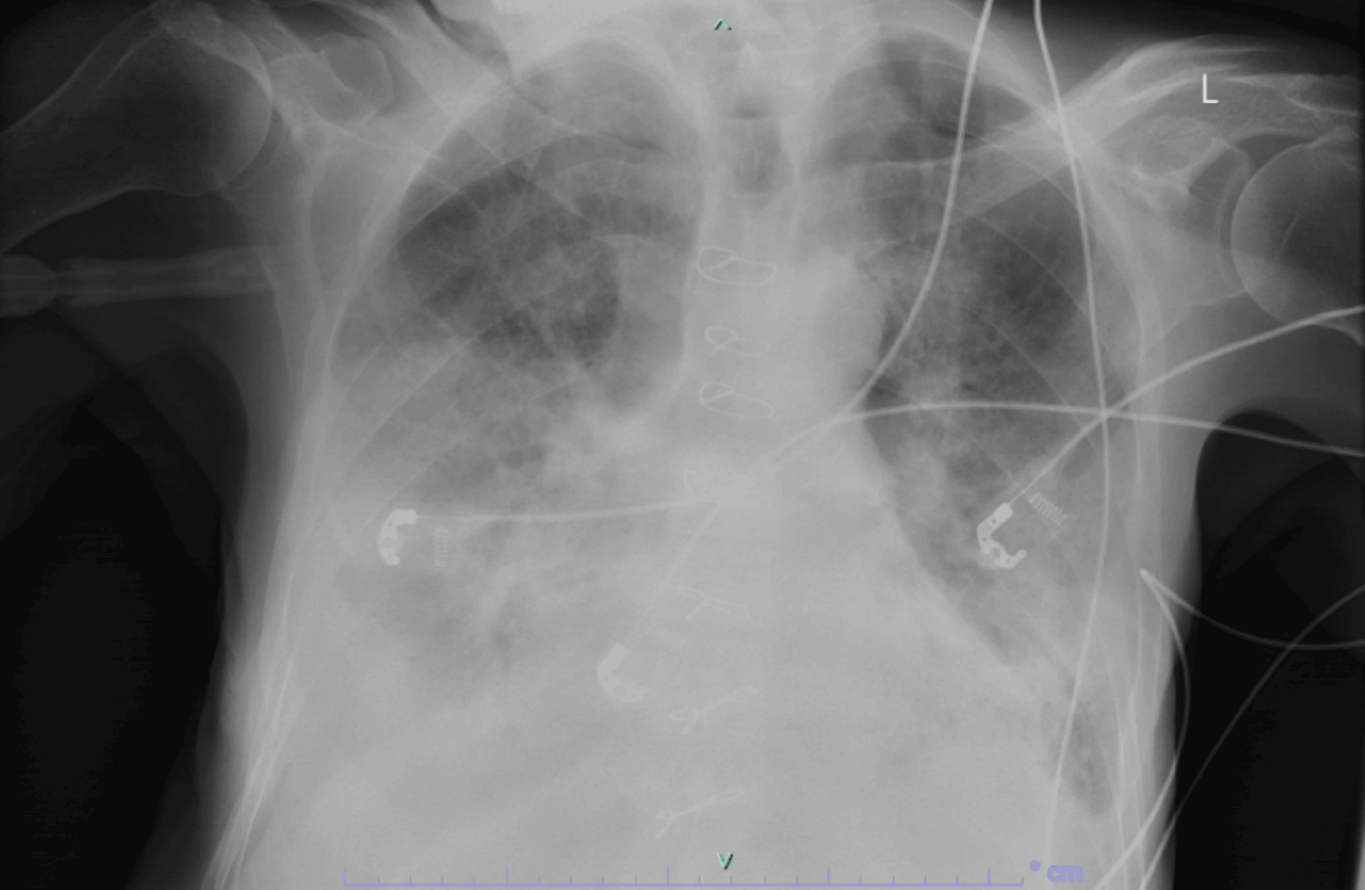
A few days later, chest X-ray deteriorated and continued to deteriorate after until he passed away, unfortunately.
